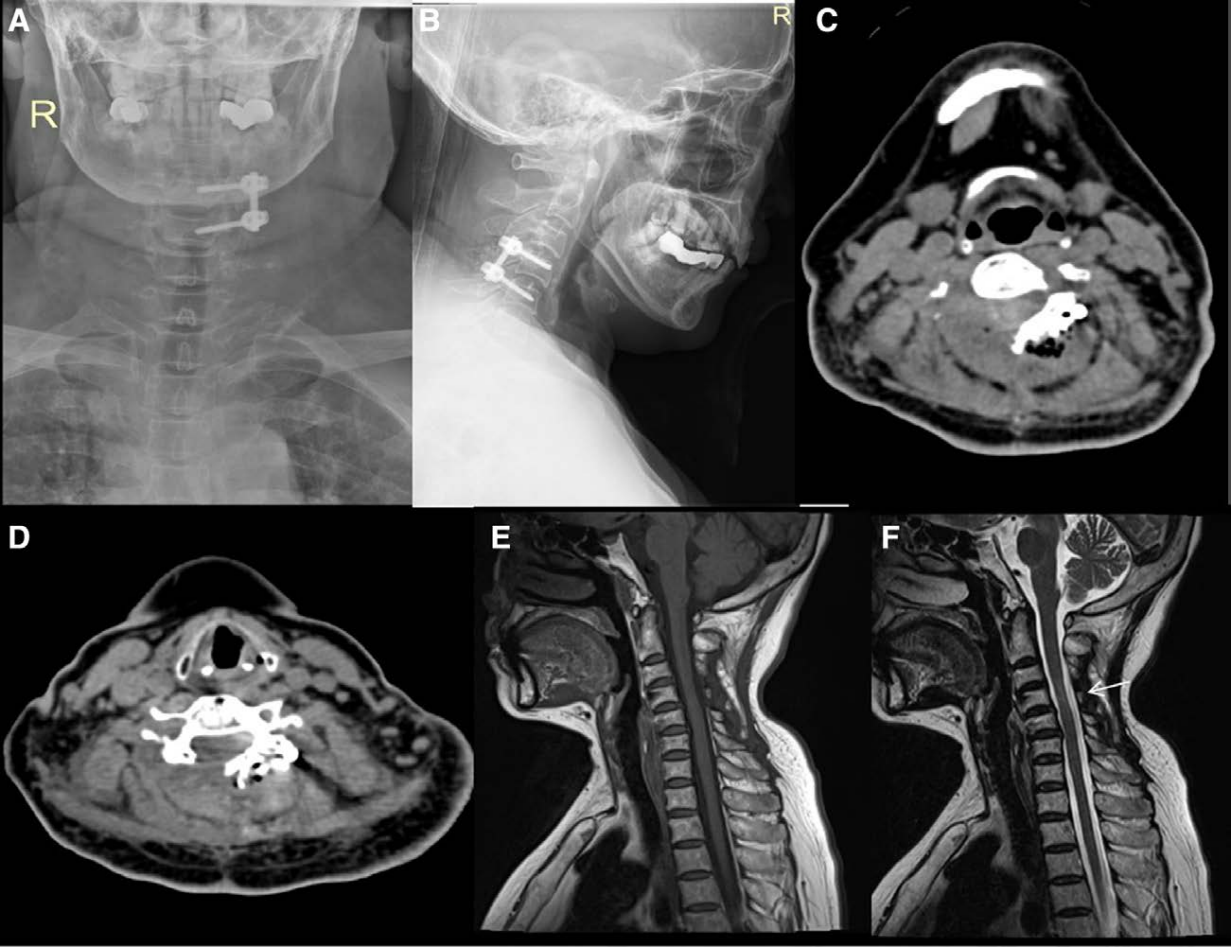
Postoperative images of the cervical spine of a 60-yr-old female. Views: (A) X-ray posteroanterior and (B) lateral positions showing that 1 screw has been inserted into the left cervical 4 and 5 pedicles, respectively, with stable internal fixation 2 wk after surgery. (C and D) Axial CT images showing no obvious bleeding in the operation area. A small amount of unabsorbed gas is visible 2 wk after the surgery. (E) Sagittal T1WI and (F) T2WI MRI showing no recurrence of the tumor 6 mo after surgery. A small amount of slightly high signal intensity is seen in the spinal cord (white arrow), suggesting there might be degeneration in the spinal cord. CT = computed tomography, T1WI = T1-weighted, T2WI = T2-weighted, MRI = magnetic resonance imaging.
